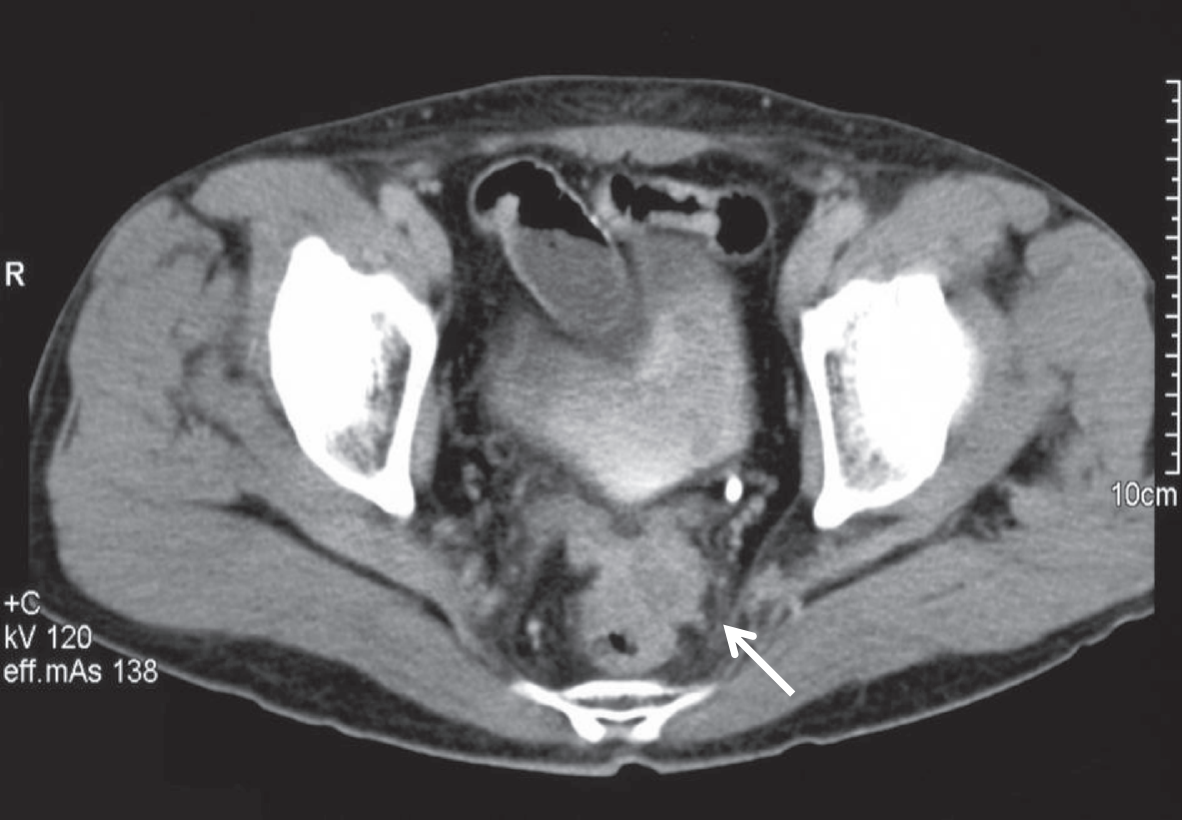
Abdominal computed tomography (CT) reveals a mass measuring 40 mm in diameter along the rectal wall and extending into the pelvis (arrow).
